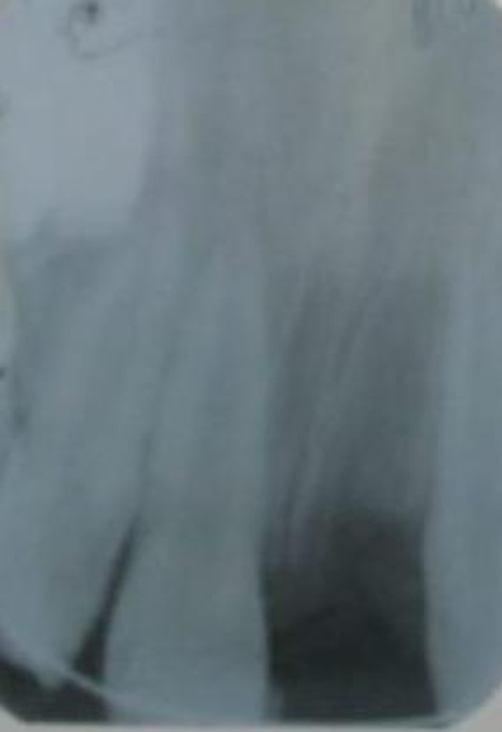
Periapical radiograph after replantation of maxillary left central incisor. Notice the orthodontic wire used for splinting.
